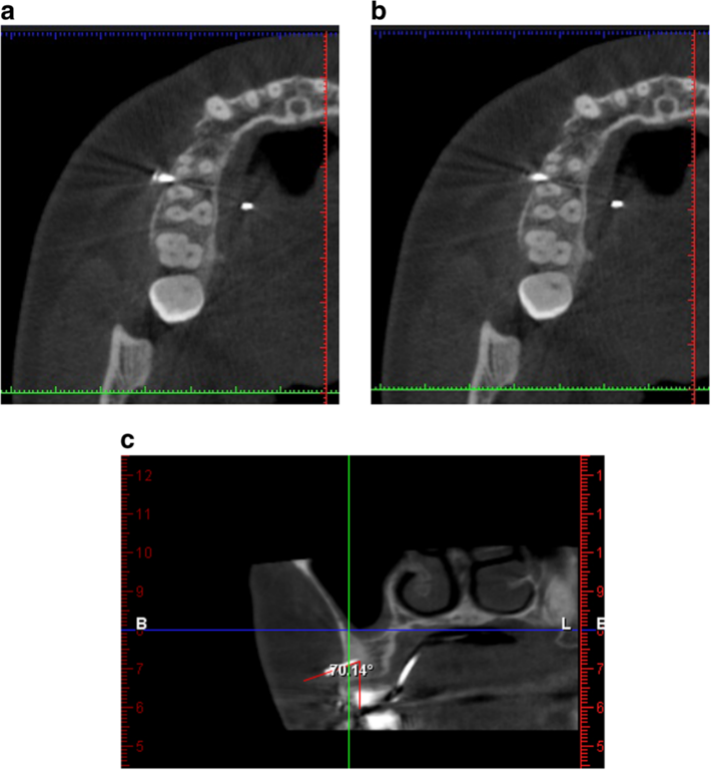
Images from cone beam computed tomography. (a to c) Cone beam computed tomography taken to check mesiodistal position and accuracy of angulation. An angulation of 70° to the vertical has been measured.
